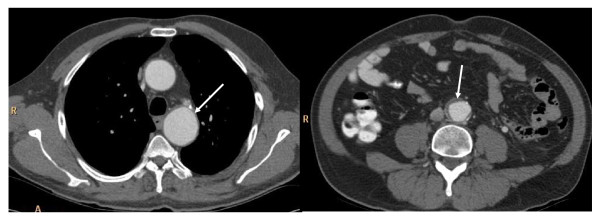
Restaging CT during follow-up. Anticoagulant therapy had been applied for 6 months. 3a: Chest CT: complete resolution of thrombus (arrow). 3b: abdominal CT: almost complete regression of endo-aortic thrombosis (arrow). Only minor thickening of aortic wall.
